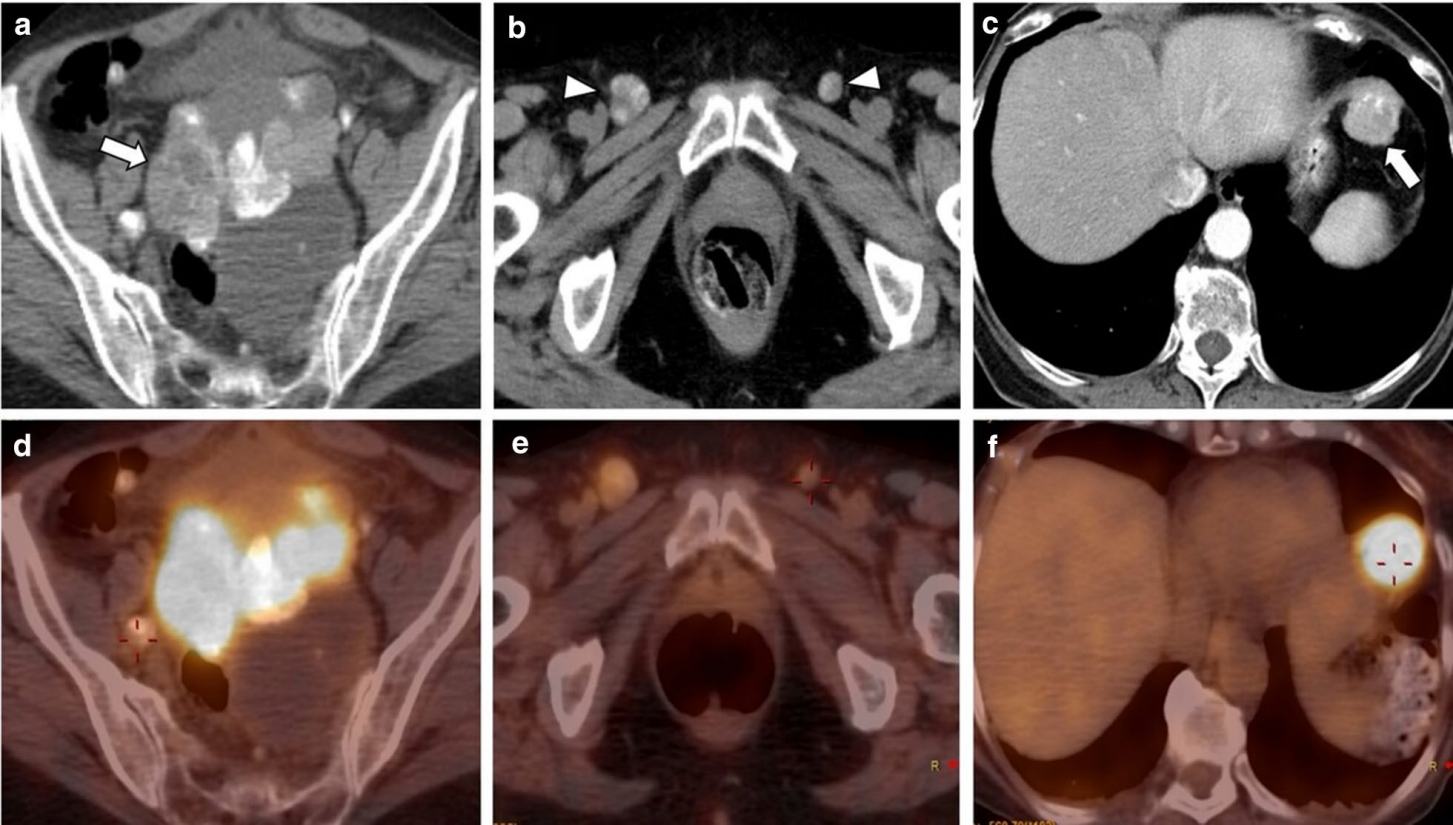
Local recurrence of LGSC with peritoneal and lymph node metastases in a 74-year-old female. Axial unenhanced CT images display a large, heterogeneous, mixed tumour in the vaginal dome (arrow) (a) with bilateral inguinal lymph node metastases (arrowheads) (b), both with calcified psammoma bodies. Axial enhanced CT image reveals a peritoneal metastasis in the left hypochondrium peritoneum (arrow) (c). FDG-PET/CT images show a hypermetabolic pelvic lesion (d), bilateral inguinal lymphadenopathies (e) and a left upper hypochondrium peritoneal lesion (f), suggestive of malignancy
